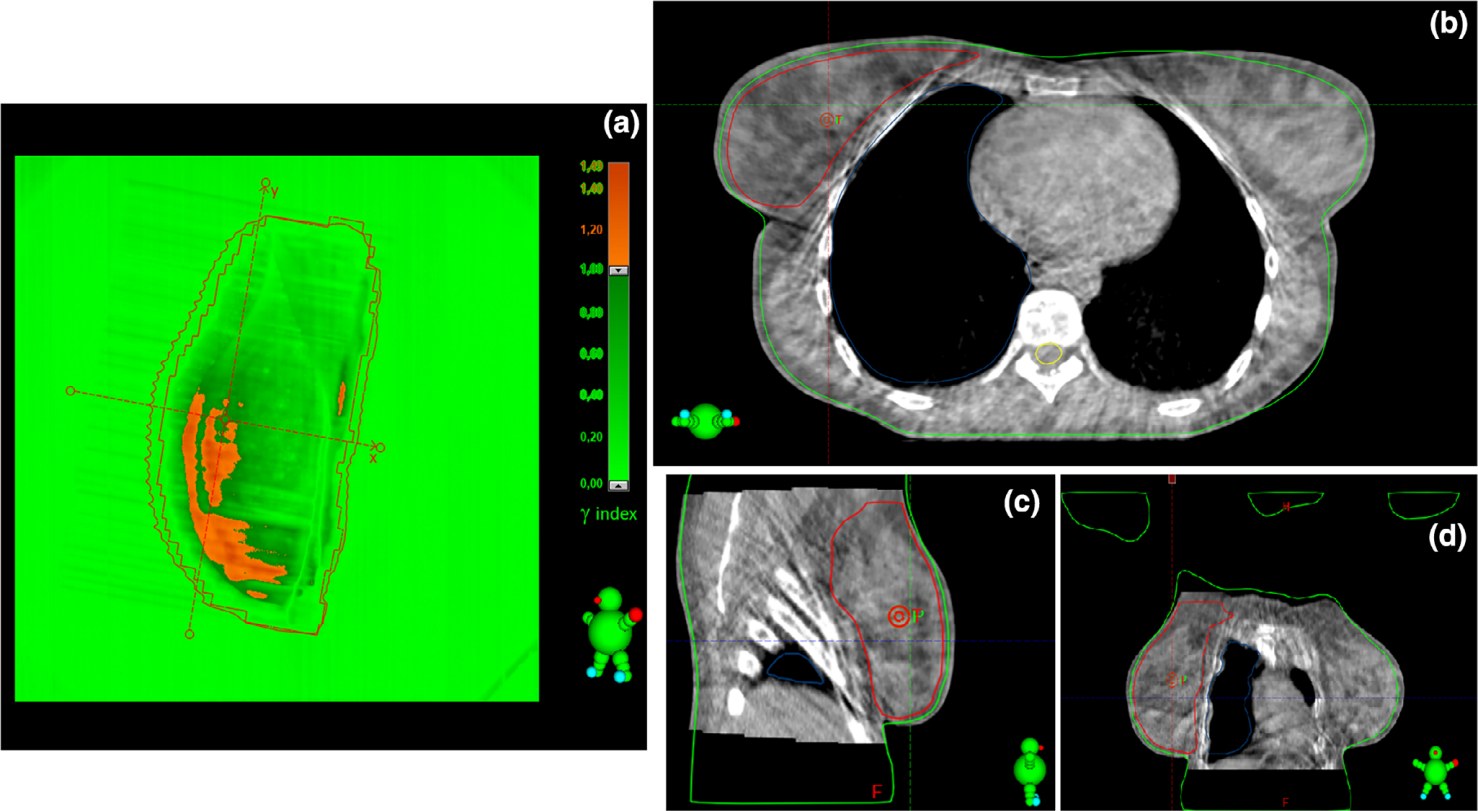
Comparison between the EPID image of the eleventh fraction and the reference fraction using PD (a), offline review of the CBCTfr_11_ vs. p‐CT comparison in the axial (b), sagittal (c), and coronal (d) planes. CBCT, cone beam computed tomography; EPID, electronic portal imaging device; PD, Portal Dosimetry; p‐CT, planning‐CT.
